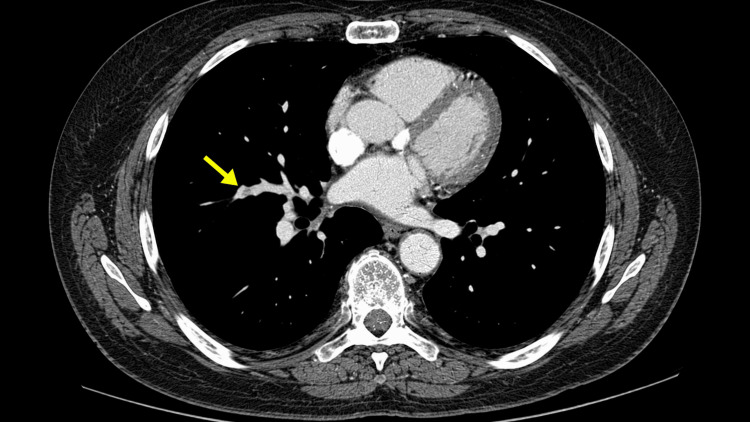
Axial CT pulmonary angiography (mediastinal window) demonstrating a filling defect within a segmental branch of the right upper lobe pulmonary artery (arrow), consistent with acute segmental pulmonary embolism. CT: computed tomography
